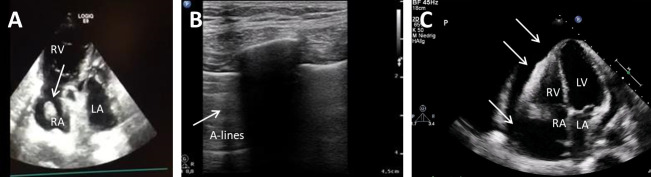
Ultrasound in obstructive shock. (A) TTE shows a
